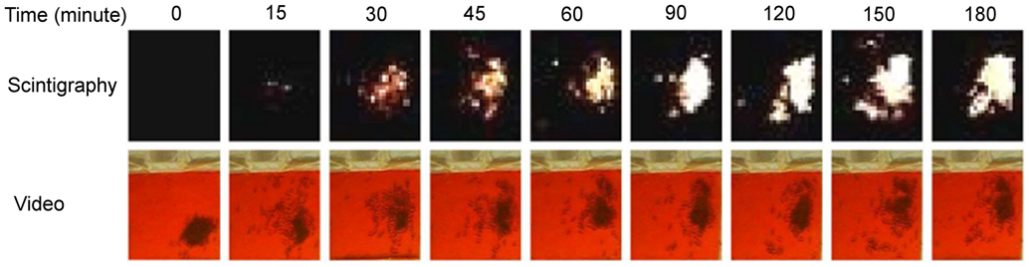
Images of the radioactivity and ants as food enters the nest. Example of pictures obtained during the three hours of observation. First row: pictures obtained by scintigraphy, second row; pictures obtained with the webcam. Nest entrance located at the bottom.
